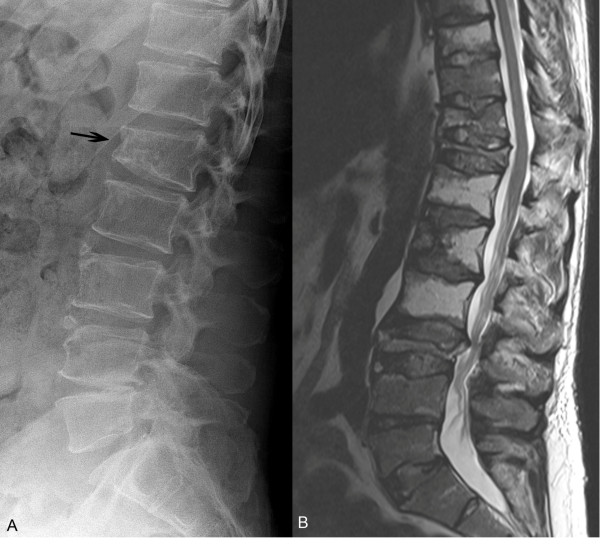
Multiple Myeloma. A. A lateral radiograph of the lumbar spine shows diffuse osteopenia and a pathologic compression fracture of the L1 vertebral body (arrow). B. A midsagittal T2-weighted MR image of the same patient obtained several months later reveals extensive marrow infiltration (low signal intensity within the marrow of multiple vertebral bodies) and numerous vertebral body compression fractures. Significant central canal stenosis has resulted from retropulsion of vertebral fragments from the pathologic fractures. Radiographs in patients with early multiple myeloma may appear normal or may exhibit only osteopenia resembling senile osteoporosis. Nuclear medicine bone scans are also frequently insensitive to multiple myeloma changes often resulting in false negative results. (Images courtesy of Lindsay J. Rowe, Newcastle, Australia).
